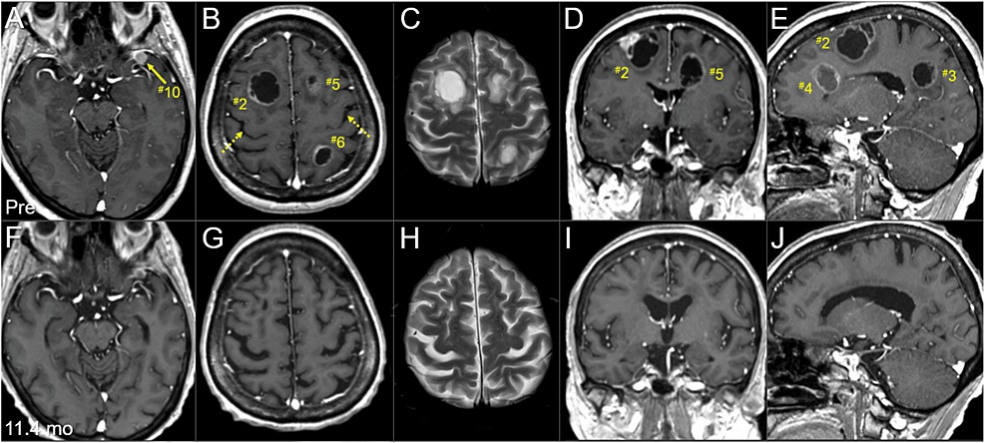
Magnetic resonance images at the diagnosis and last examination, mainly focusing on the boost lesions in the right hemisphere. The images show CE T1-WIs (A, C, E, G, I, K); T2-WIs (B, D, H, J); axial images (A-C, F-H); coronal images (D, I); sagittal images in the right hemispheres (E, J); three days before the initiation of WBRT (pre) (A-E); and 11.4 months after the WBRT initiation (F-J). (A-J) These images are shown at the same magnification and coordinates under co-registration and fusions based on the pre-irradiation images (A, F: the same cross-section; B, C, G, H: the same section). (A-E) Six lesions are observed, most of them cystic, with some solid components (^#^2,4,5). An extra-parenchymal dura-based lesion (#10) is solid. (B) The central sulci are indicated by dashed arrows. (E) Two large lesions (^#^2,4) in the right frontal lobe are in close proximity. (F-J) At 11.4 months, all the seven lesions had remarkably regressed or almost disappeared. CE: contrast-enhanced; WIs: weighted images; WBRT: whole-brain radiotherapy
